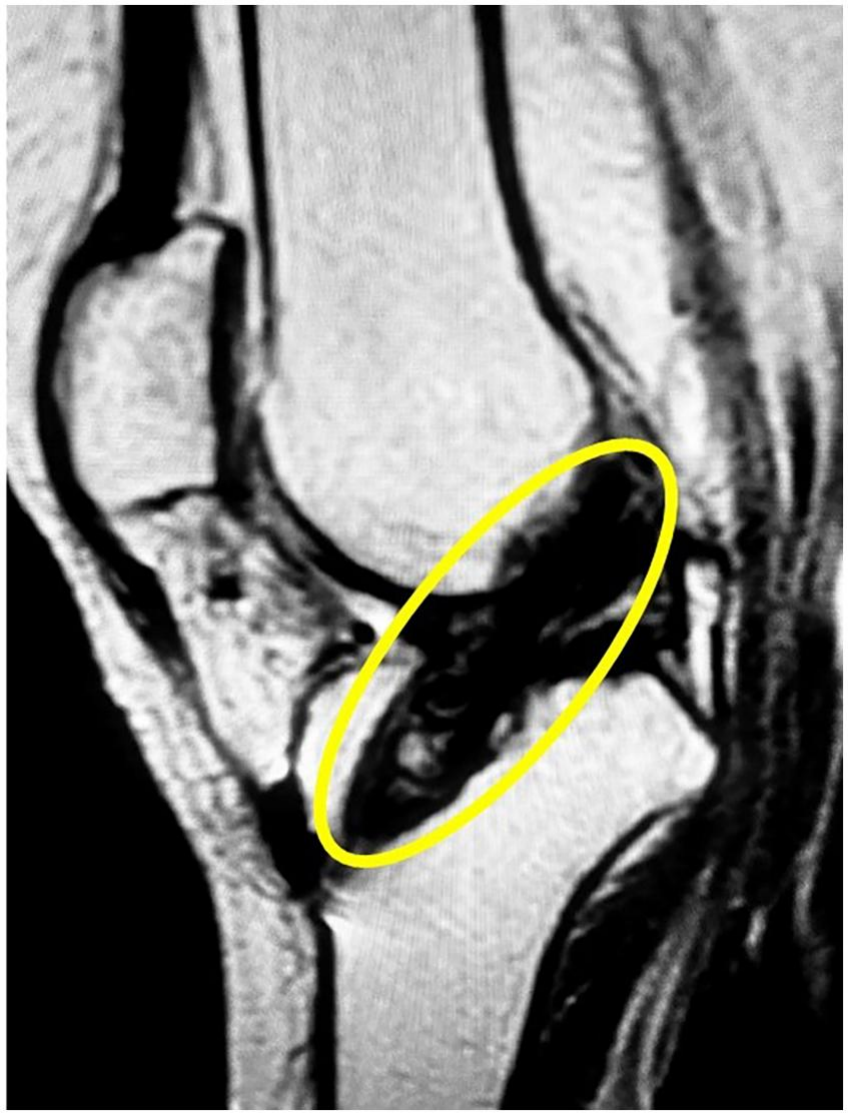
Post-operative MRI demonstrating successful ACL reconstruction with intact graft alignment.
